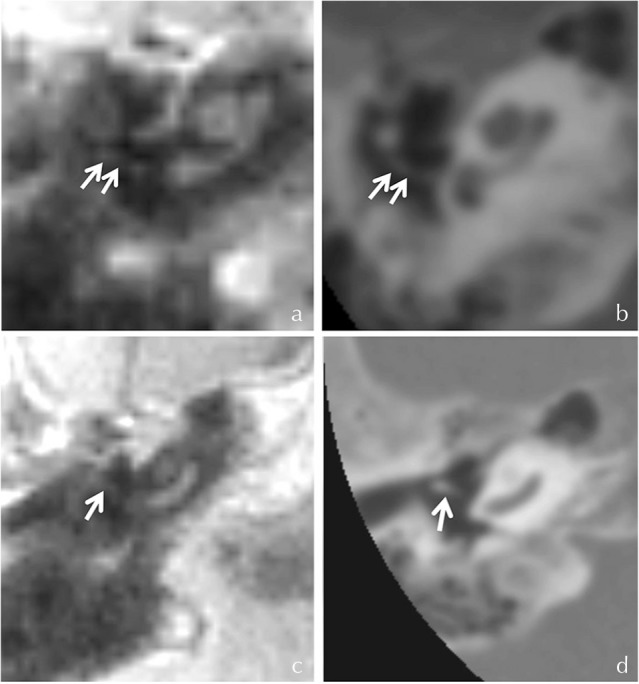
A 74-year-old woman. The long process of the incus (arrows) in the right middle ear is faintly visualized with a signal intensity between labyrinthine fluid and air on this PETRA image (a) which corresponds to the CT image (b). The manubrium of the malleus (arrow) in the right middle ear is faintly visualized with a signal intensity between labyrinthine fluid and air on this PETRA image (c) which corresponds to the CT image (d). CT, computed tomography; PETRA, pointwise encoding time reduction with radial acquisition.
